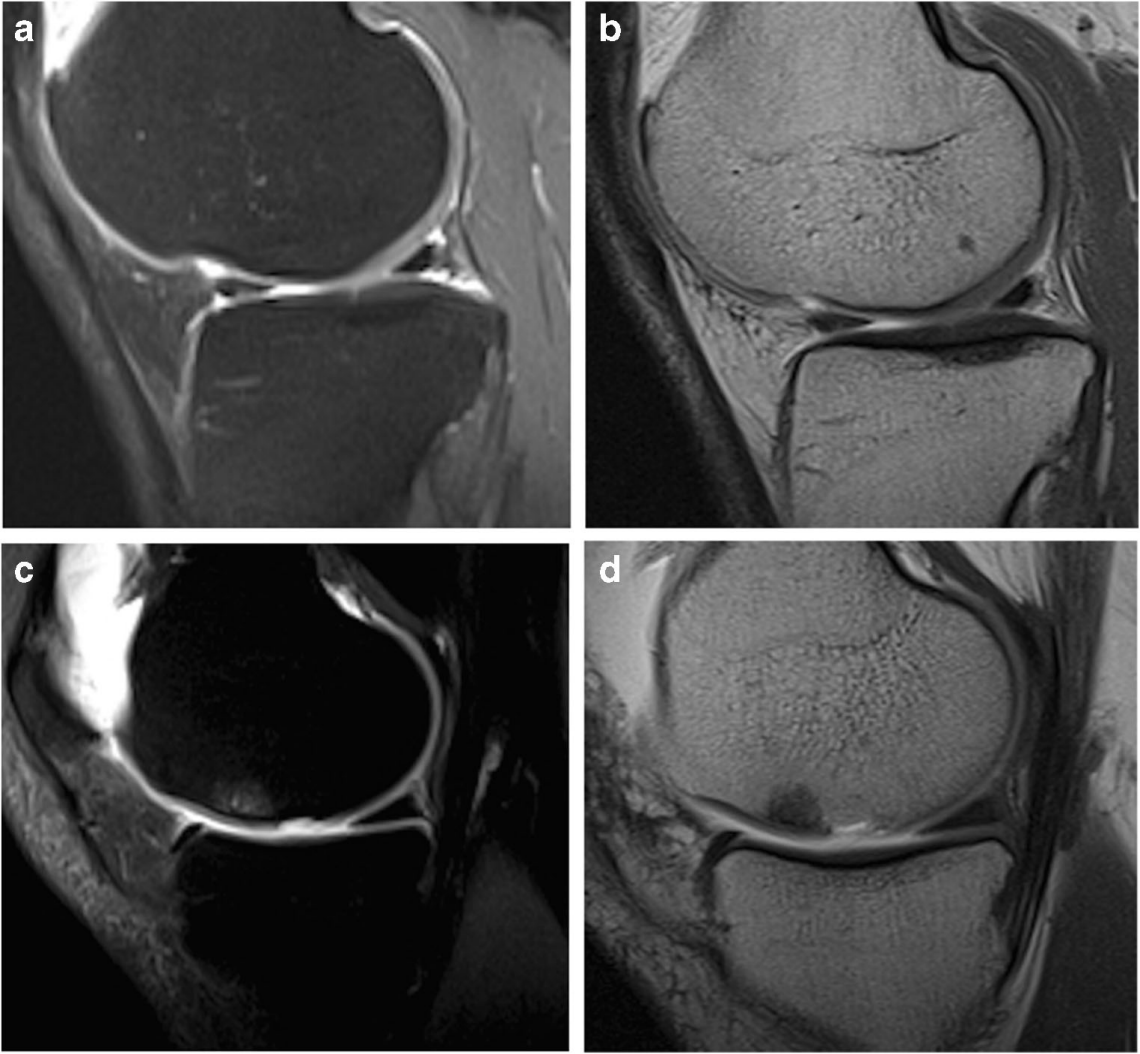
a, b A 28-year-old man treated with MACI of the internal tibial plate at long term with mMOCART score of 25, Cincinnati score 9. a Sagittal oblique FSE PD fat-saturated image shows structural incongruence of the chondral surface (arrowhead). b Sagittal oblique SE T1-weighted image. Contrast enhancement of the chondral implant (arrowhead). c, d A 47-year-old man treated with MACI of the internal femoral condyle at long-term with mMOCART score of 20, Cincinnati score 5. c Sagittal oblique FSE PD fat-saturated image shows structural incongruence of the chondral surface (arrow) and the presence of subchondral bone oedema with incomplete integration to the border zone. d Sagittal oblique SE T1-weighted image. Contrast enhancement of the chondral implant (arrow) [images obtained with “Unit1”]
